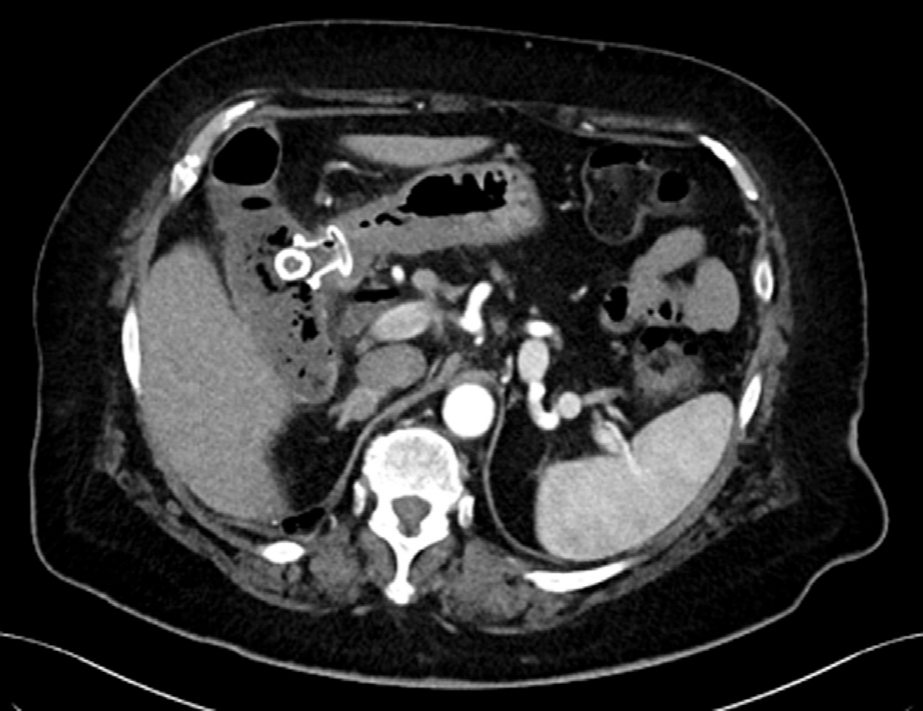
CT scan image of a 15-mm stone impacted in the distal flange of the lumen-apposing metal stent.
